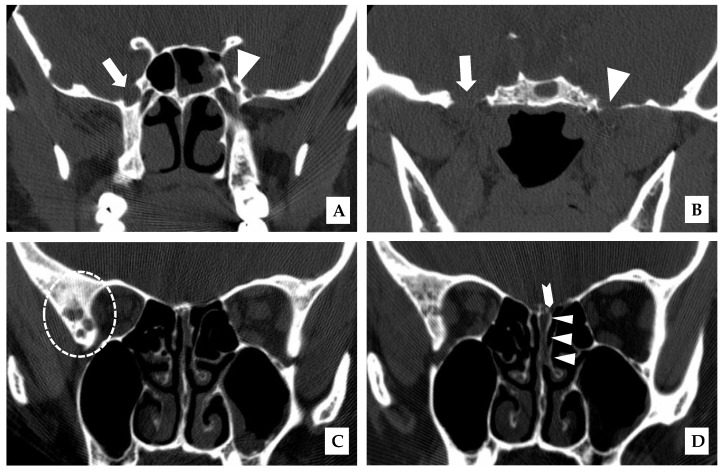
Coronal plane, unenhanced CT scan of the paranasal sinuses obtained with bone window and in prone position. (A–D, Dec. 2007). (A): prominent right (arrow) and normal left (triangle) foramen rotundum; (B): prominent right (arrow) and normal left foramen ovale (triangle); (C): prominent right sphenoidal arachnoid pits (dashed circle); (D): focal defect of the left planum sphenoidale (chevron) and adjacent opacification of the left ethmoid sinus and upper nasal cavity (CSF leak, triangles).
